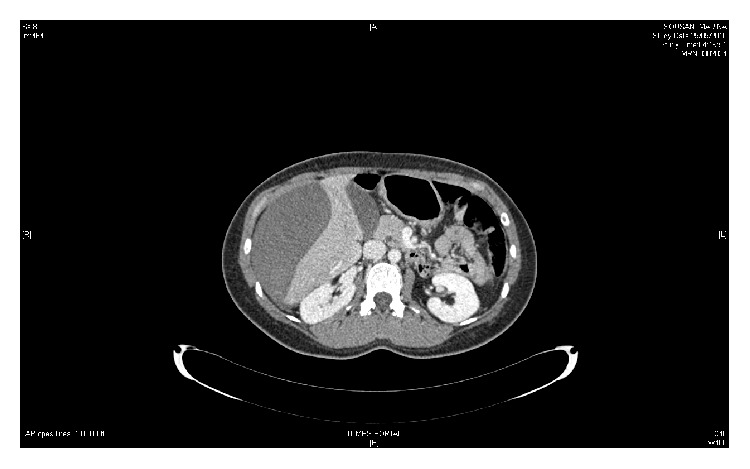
Abdominal CT scan with subcapsular hematoma and active bleeding.
